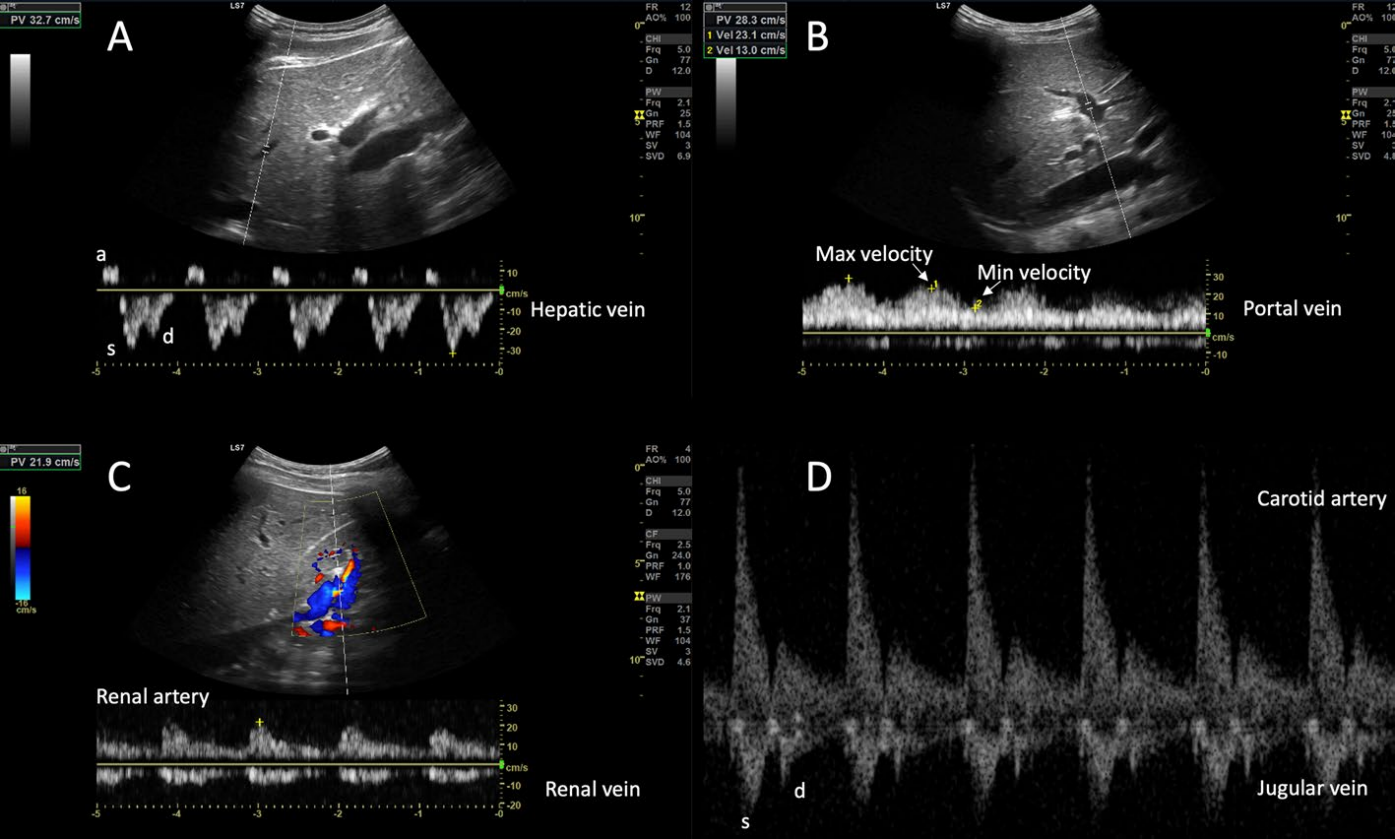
Example of venous scoring during high preload condition. A The hepatic vein Doppler at the bottom with labeled a, s and d waves showing an S > D morphology. B The portal vein showing the maximum and minimum velocities use to calculate the portal pulsatility index. C The renal artery and vein revealing a ‘pulsatile fused’ or ‘short end-diastolic pause’ morphology. D The carotid artery and jugular venous spectra from the wearable Doppler. The jugular has an S > D morphology most comparable to the hepatic vein
